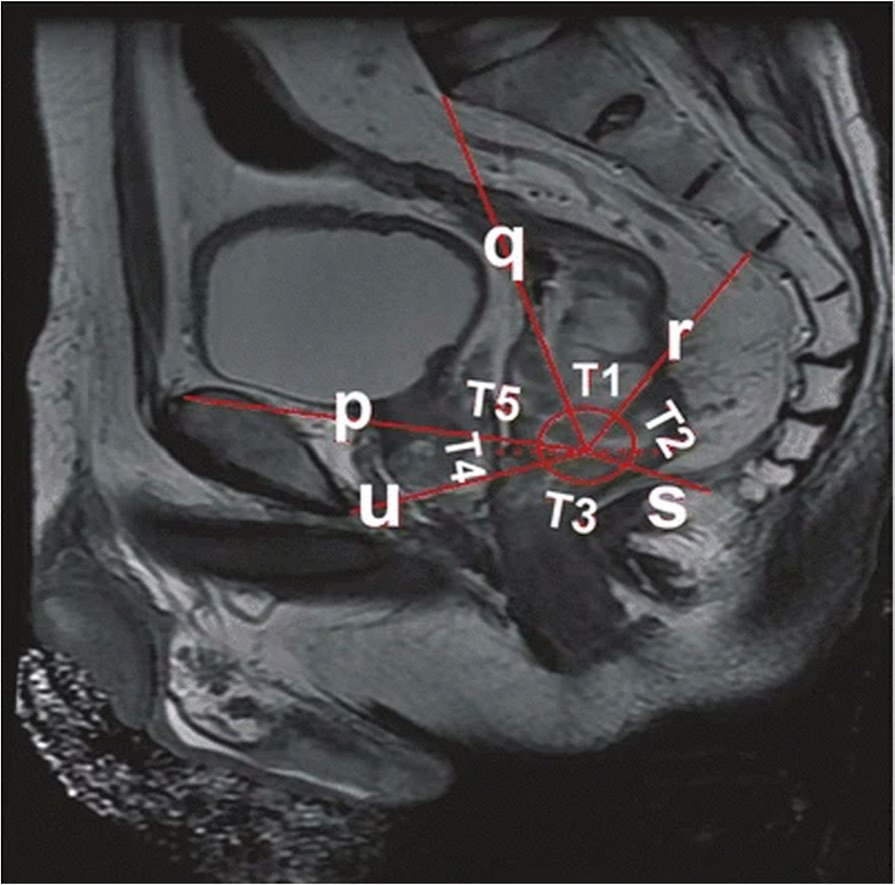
Sagittal magnetic resonance image of the pelvis: l T4 angle, i.e., the angle between the upper and lower borders of the pubic symphysis with the lower border of the tumor as the vertex [31]
